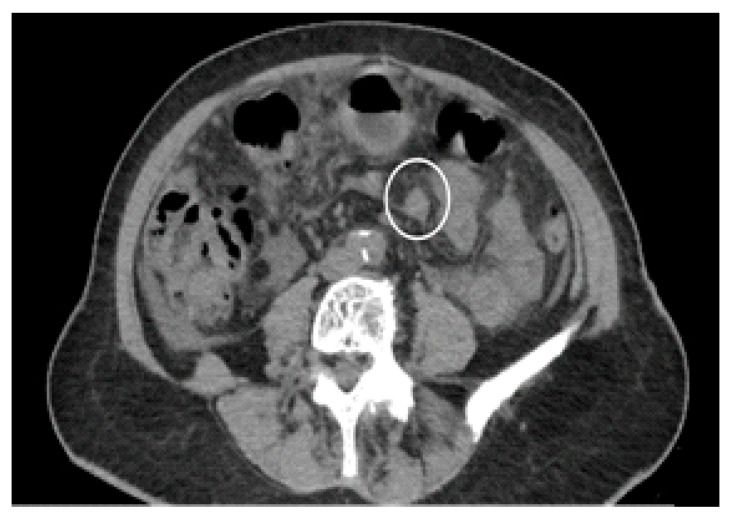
Contrast-enhanced CT scan, axial views. Case of gastric adenocarcinoma in a female, 79 y. Multiple nodules (white circle), localized in the mesogastric and ipogastric area on the anterior abdominal wall, between bowel loops and in pelvis.
